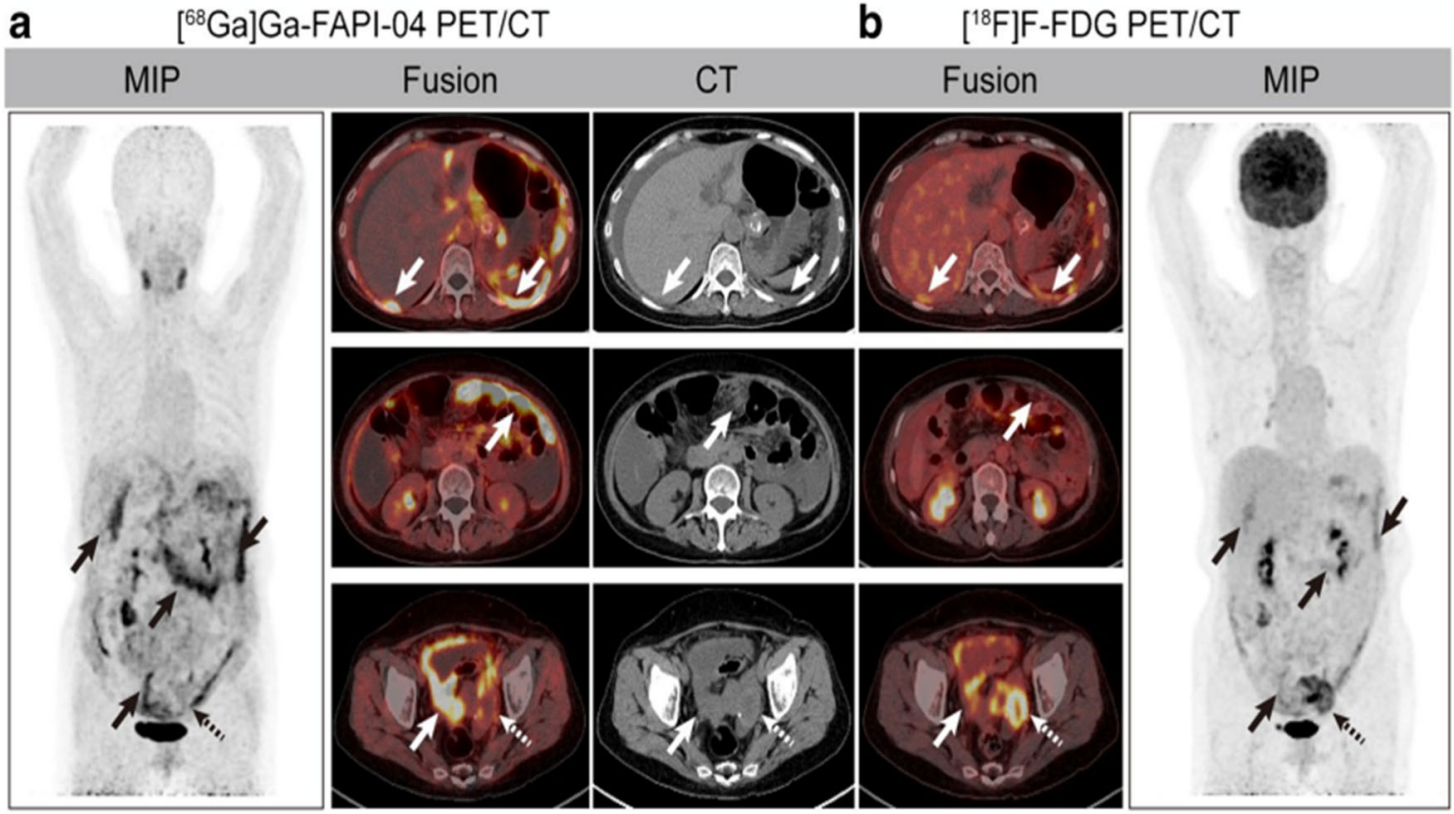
A 66-year-old woman underwent preoperative staging after being diagnosed with left ovarian high-grade serous carcinoma. ^68^Ga-FAPI-04 PET/CT demonstrated mild focal uptake (SUVmax, 4.5) in the left primary tumor ((a); dotted arrow head) and distinctive uptake (SUVmax, 9.7) in widespread peritoneal metastasis ((a); solid arrowhead). ^18^F-FDG PET/CT revealed high focal uptake (SUVmax, 8.8) in this cystic-solid ovary tumor ((b); dotted arrowhead) and slight and diffuse uptake (SUVmax, 2.5) in the peritoneal metastases ((b); solid arrowhead). For this representative participant, ^68^Ga-FAPI-04 PET/CT detected more metastatic lesions compared with ^18^F-FDG PET/CT regarding the peritoneal metastases. [From ref. ^113^].
